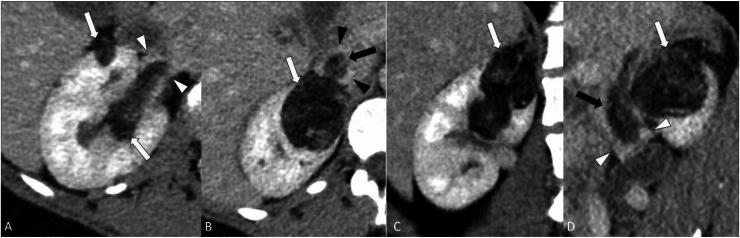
Contrast-enhanced CT of the right kidney demonstrating on axial image (A) several fat-containing renal masses (white arrows) with extension of one lesion into the renal vein (white arrowheads). On a more superior axial image (B) a dominant fat containing renal mass is seen (white arrow) with a corresponding fat containing lesion (black arrow) in the adjacent inferior vena cava (black arrowheads). On the coronal reformatted image (C) the superior-to-inferior extent of the dominant lesion is again seen (white arrow). On the sagittal view (D) the dominant lesion is present (white arrow) with extension of fat-density material (black arrow) into the right renal vein and IVC (white arrowheads).
